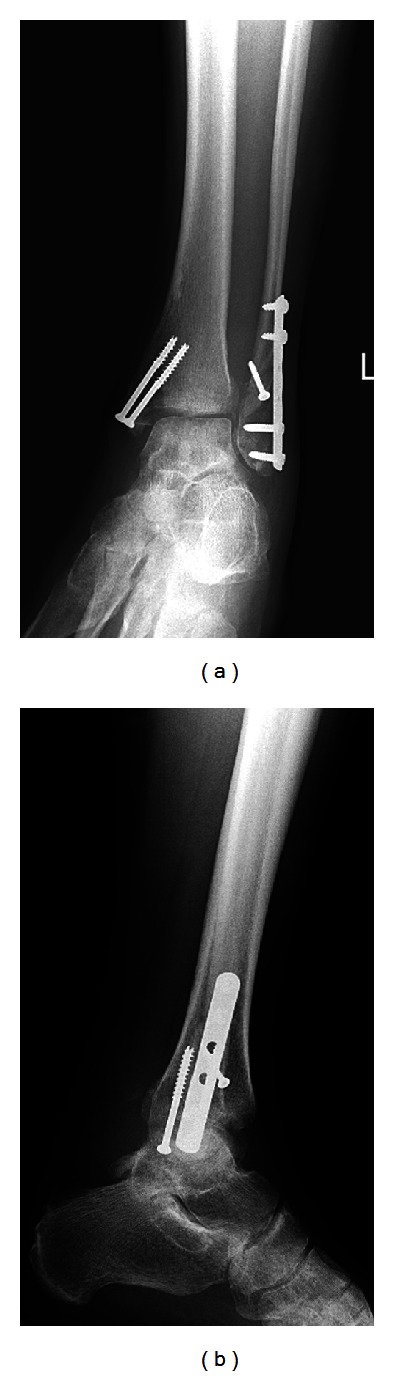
Postoperative mortise and lateral radiographs taken three months after surgery.
